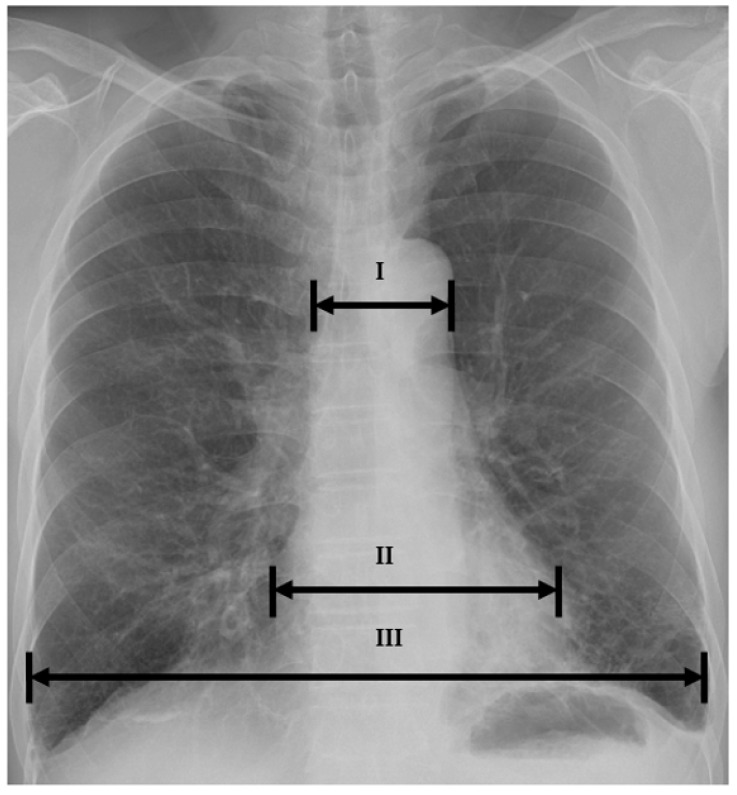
Calculation of radiologic parameters on simple chest radiography. Cardio-thoracic ratio = II/III; mediastinal-thoracic ratio = I/III; mediastinal-cardiac ratio = I/II; I = mediastinum width; II = maximal transverse diameter of heart; III = maximal transverse diameter of chest.
